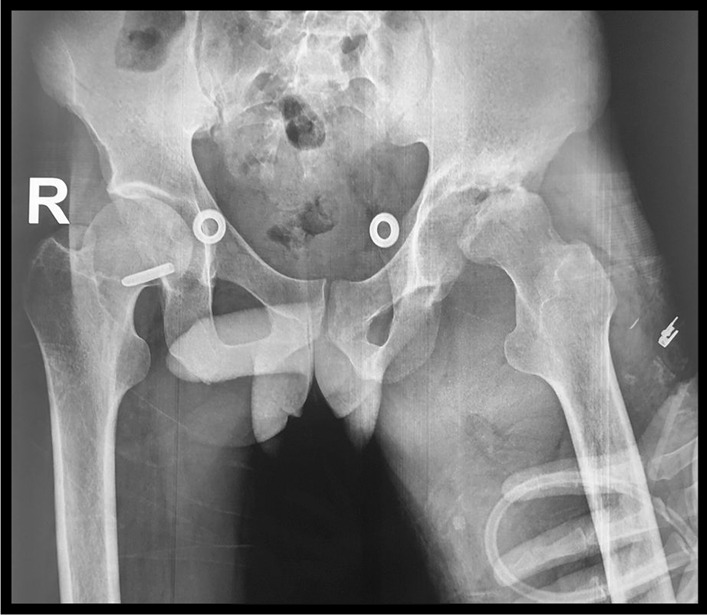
Anteroposterior radiograph showing the pelvis
